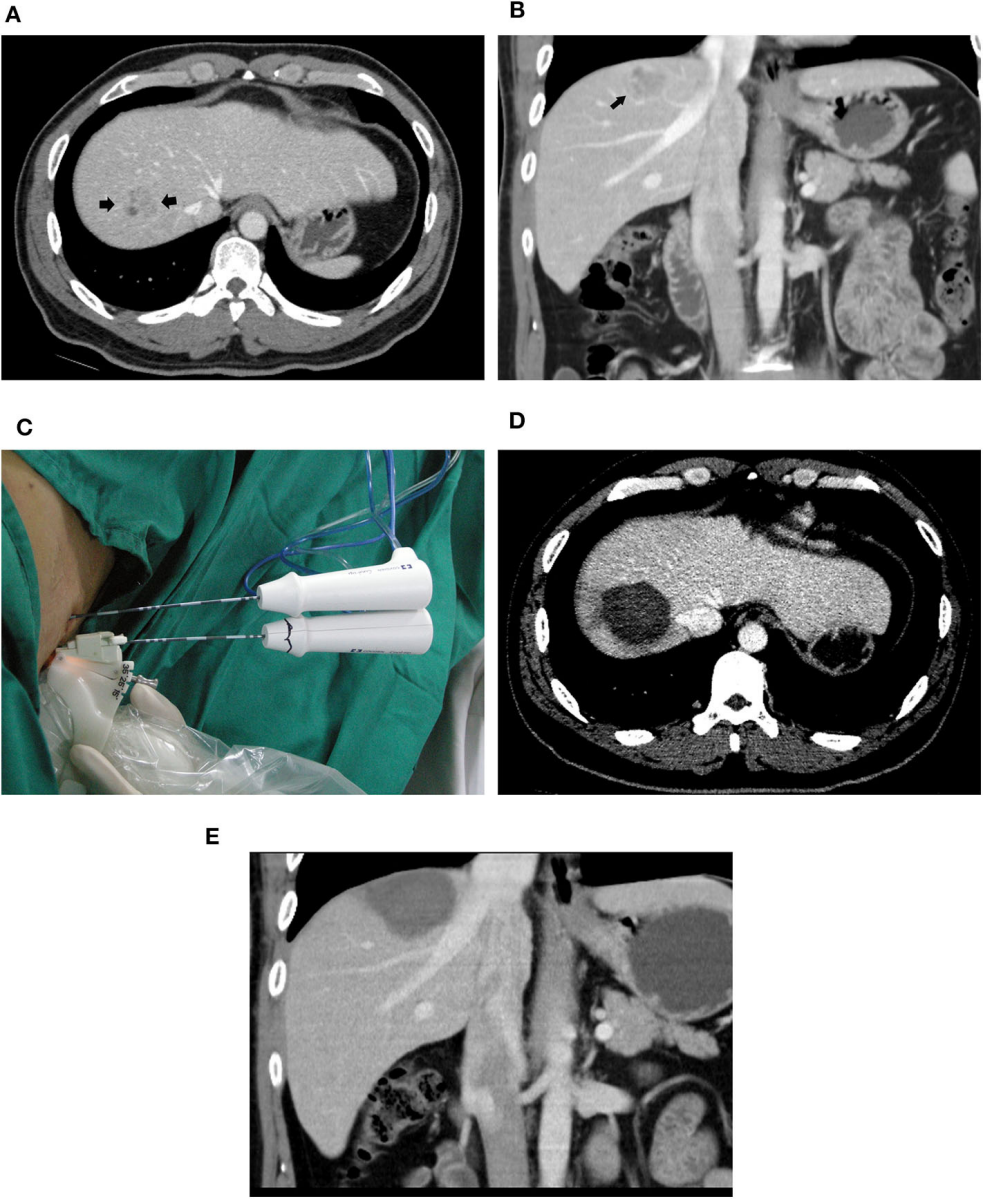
A 39-year-old male patient with HCC who underwent radiofrequency ablation with a multiple-electrode switching system. Pre-ablation CT scans showed a 2.6-cm tumor (arrowhead) in the segment VIII of the liver in the transverse (A) and coronal (B) view. (C) Ultrasound-guided RFA using the multiple-electrode switching system was performed with two RF electrodes for 16 min. CT scans obtained 1 month after ablation showed that the tumor was completely ablated in the transverse (D) and coronal (E) view.
